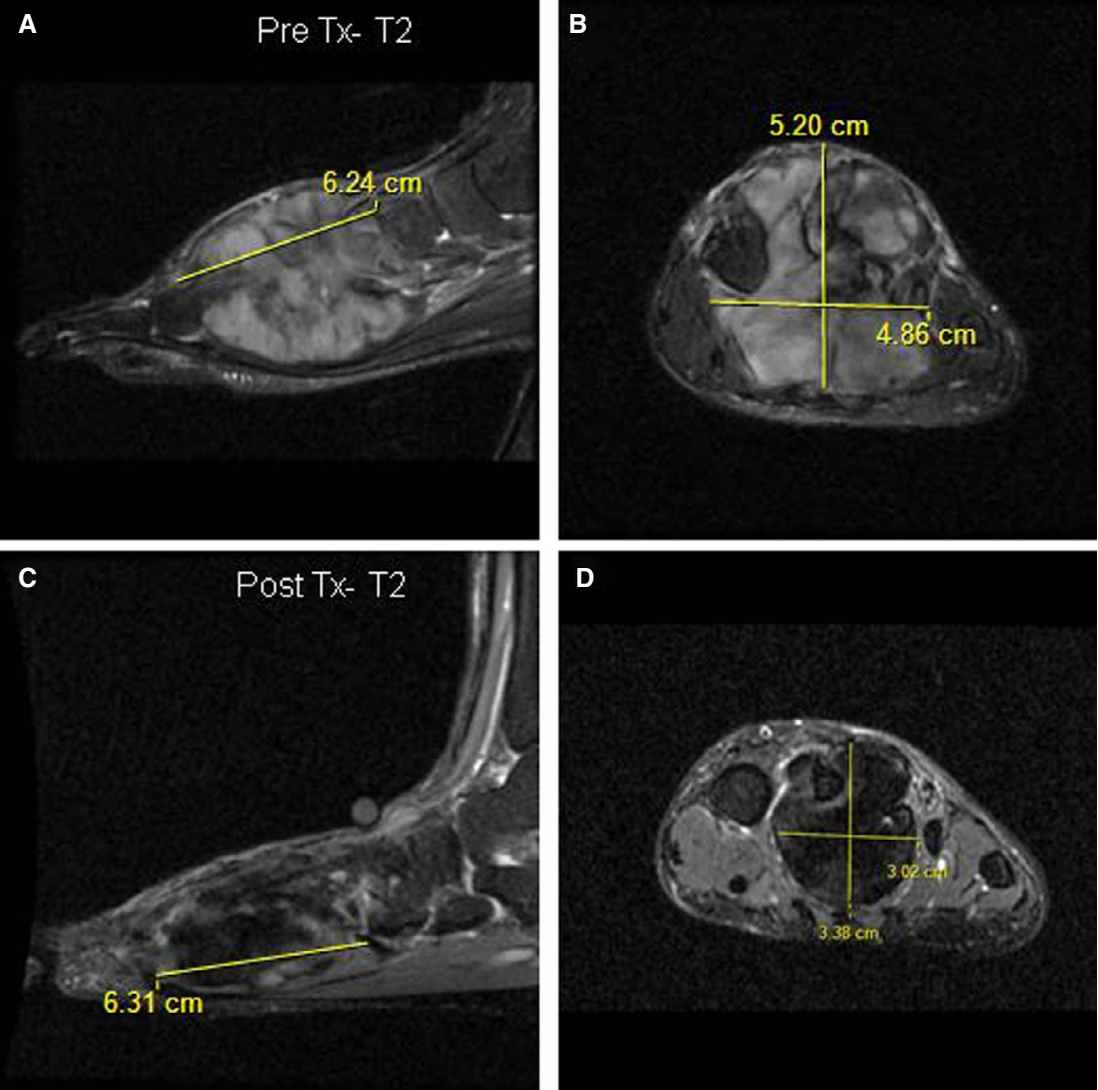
MRI imaging showing T2 characteristics of DF in foot in response to MTX/VNL. T2‐fat‐saturated sagittal and coronal MRI images of DF in left foot pre‐ (A and B) and post‐ (C and D) MTX/VNL. No response to treatment (Tx) as per *D*
